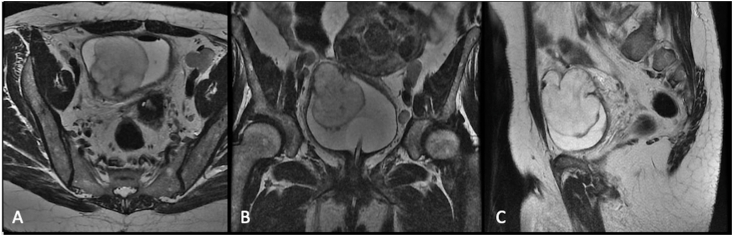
MRI of the Pelvis in the T2 phase in 3 incidences: A) Axial, B) Coronal and C) Sagittal – evidencing injury to the right posterior face of the bladder surpassing the muscular layer and serous of the bladder with intraperitoneal invasion of about 23 mm. Lesion measurements 62 × 53 × 54 mm. Contact of the upper face of the lesion was noted for about half of the adjacent parts of the round ligament.
